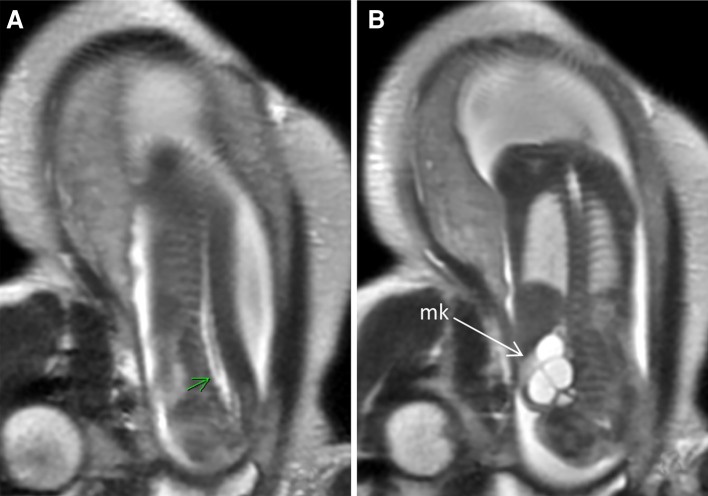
Prenatal MRI of a patient with cloaca. a
